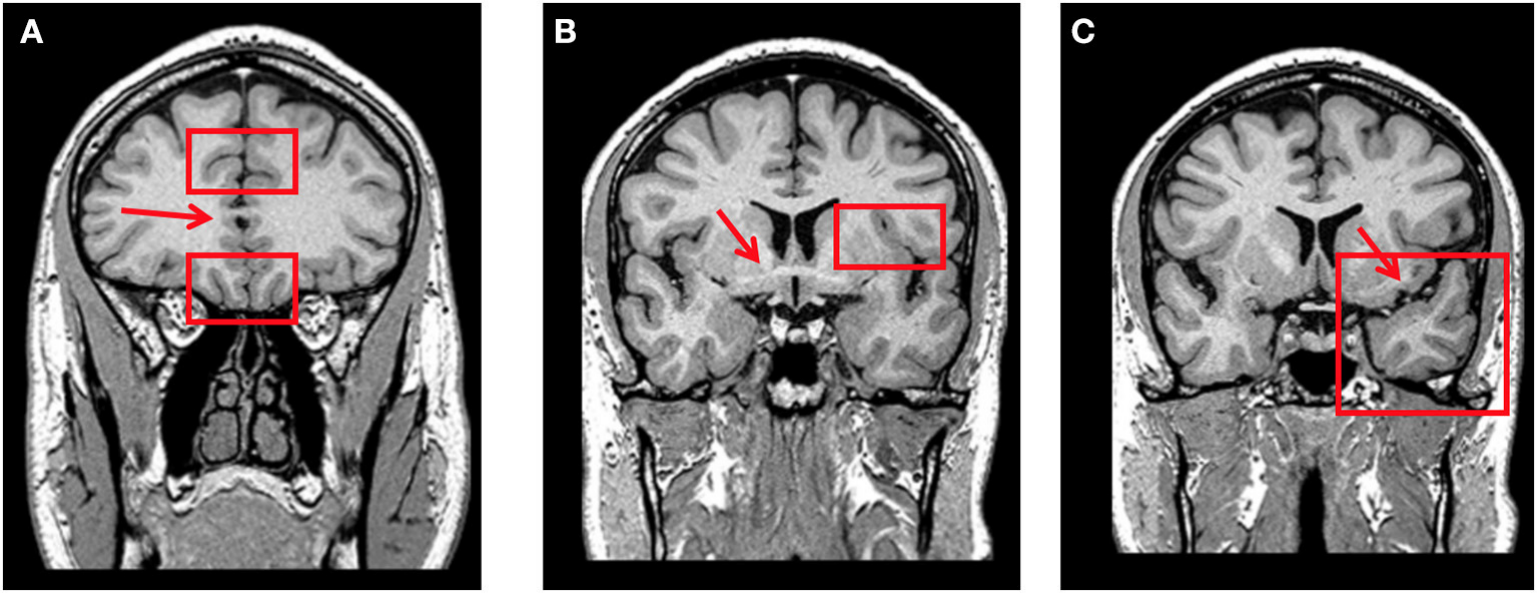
(A) Coronal T1-weighted MRI at the level where the corpus callosum is first visible (red arrow). Orbitofrontal cortex is rated through the olfactory sulcus (lower red rectangle) and rostral anterior cingulate cortex through the cingulate sulcus (upper red rectangle) on this slice. (B) Coronal T1-weighted MRI at the level where the anterior commissure is first visible (red arrow). Fronto-insular cortex is rated through the circular sulcus (left-side red rectangle) on this slice and the two posterior. (C) Coronal T1-weighted MRI at the level where the connection between the frontal and temporal lobes is no more visible (left-side red arrow). Anterior temporal cortex is rated in this slice (left-side red rectangle).
